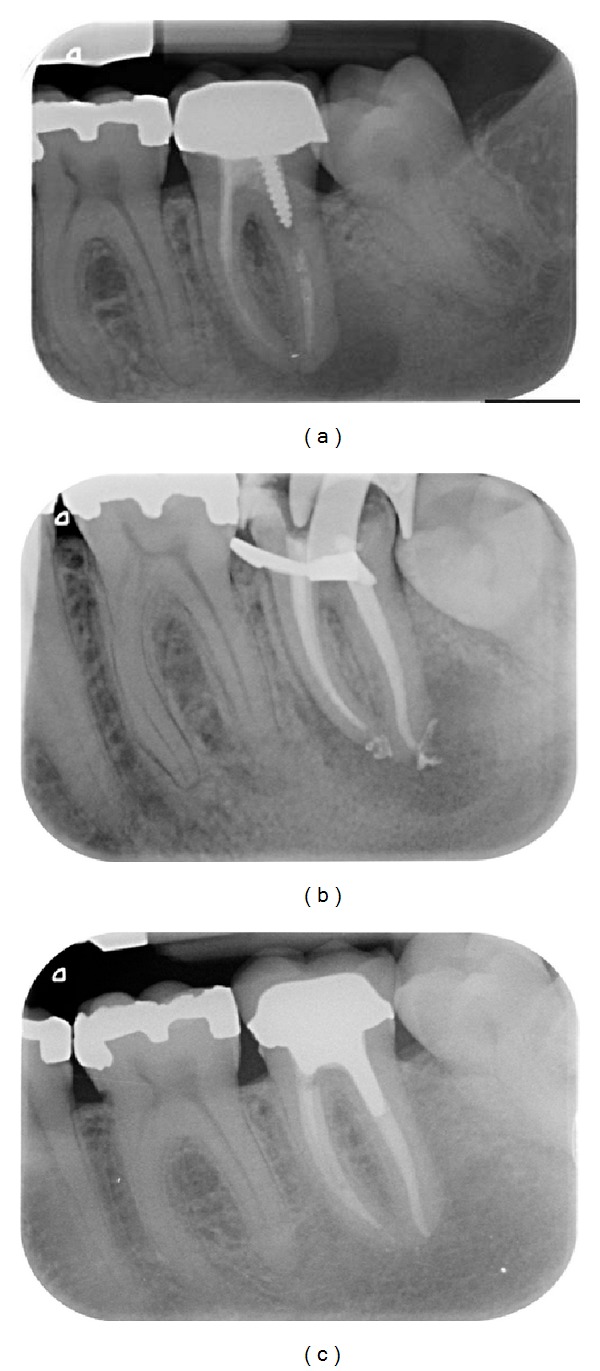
Case classified as healed. (a) Preoperative view of the 37, painful, with failure of an initial endodontic treatment performed more than one year ago. Note the apical radiolucency (PAI = 5). (b) Periapical X-ray control immediately after the endodontic retreatment. (c) Recall after 4 years. Note the disappearance of the apical radiolucency. This tooth is classified as “healed”: disappearance of pain and functional and asymptomatic tooth with complete healing of periapical lesion.
